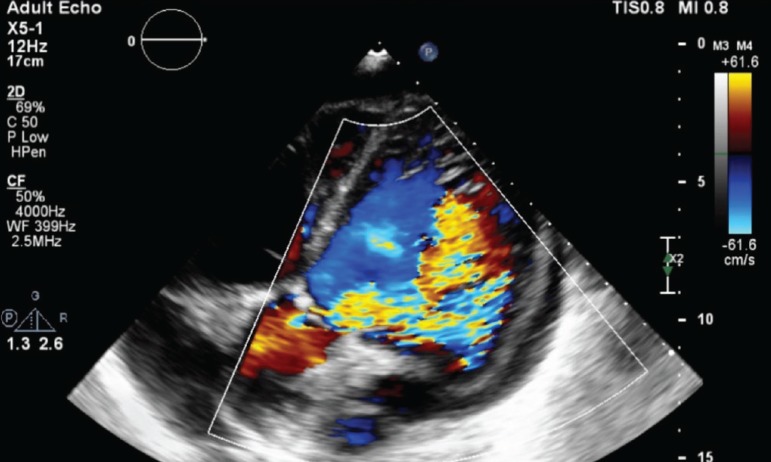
Transthoracic echocardiogram demonstrating severe aortic
